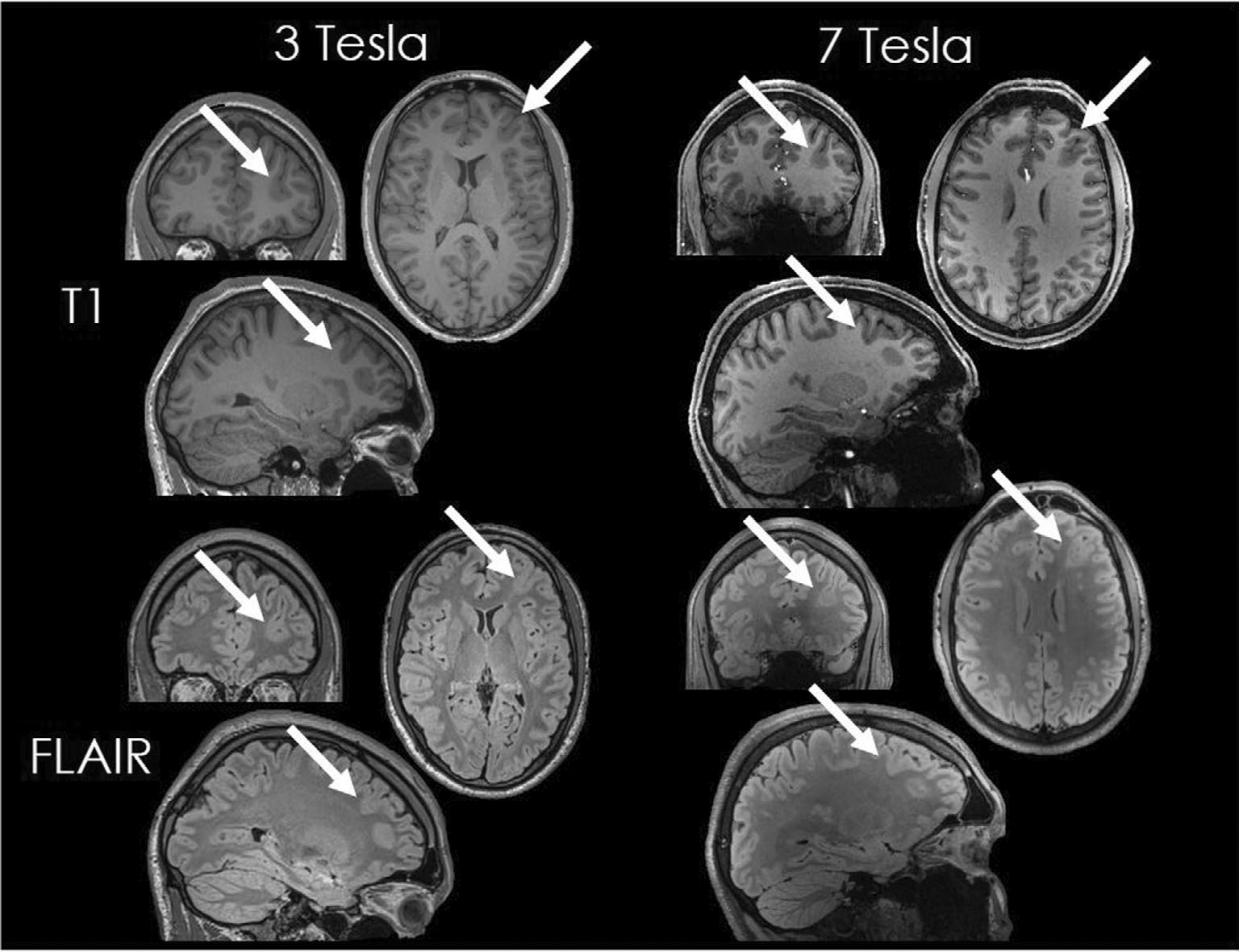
3D‐T1 and 3D‐FLAIR weighted sequences using 3 Tesla (left images) and 7 Tesla (right images) of a 40‐year‐old male patient with focal cortical dysplasia in the left frontal region.
